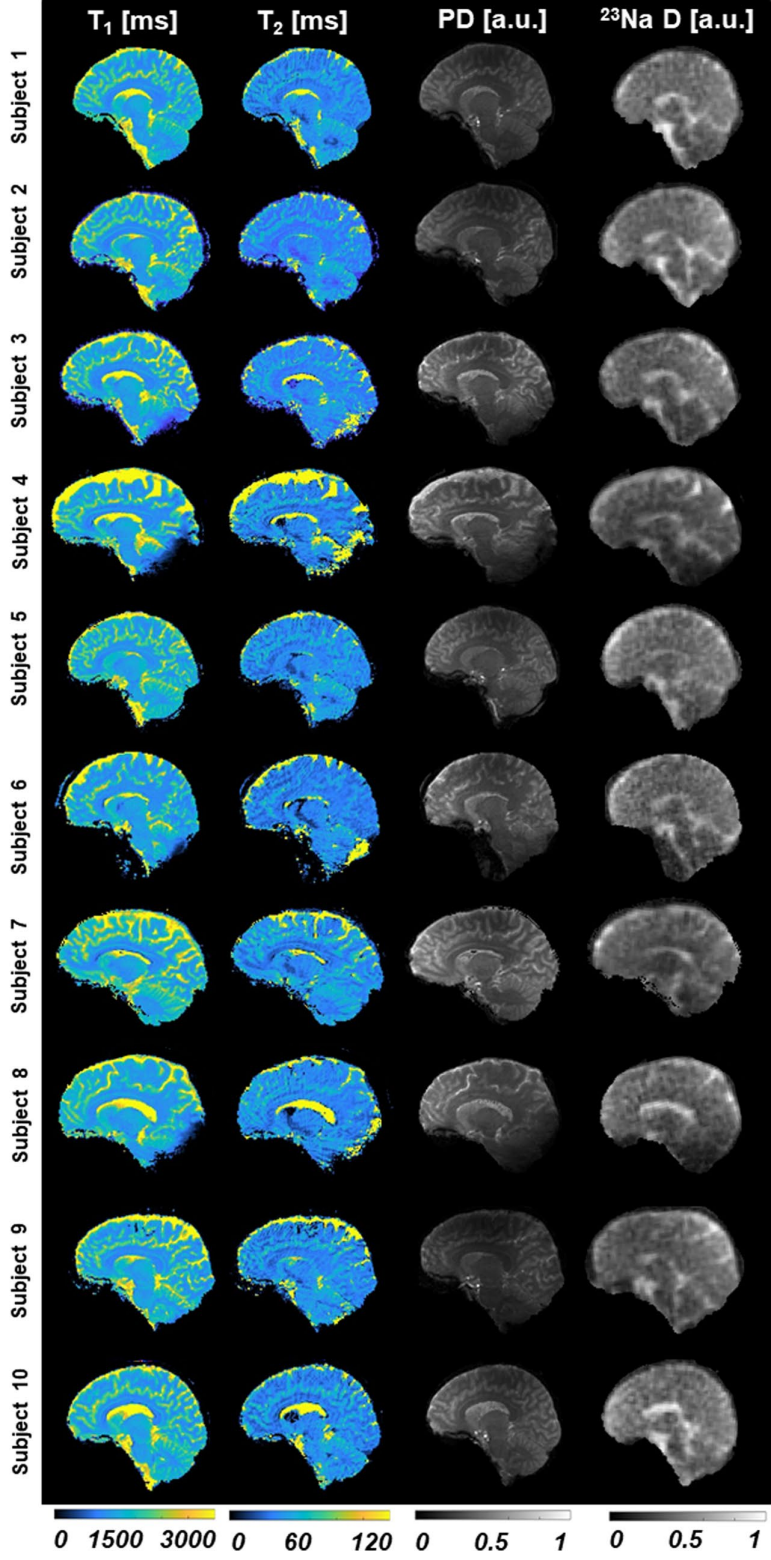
Maps from the first scan acquired in all the subjects (after co-registration, using subject 1 as reference) with simultaneous ^1^H MRF/^23^Na MRI. The in-plane resolution is 1.5 × 1.5 mm^2^ for the proton images and 2.85 × 2.85 mm^2^ for the sodium image. Slice thickness is 3 mm for both nuclei. PD is normalized proton density and ^23^Na D is normalized sodium density.
